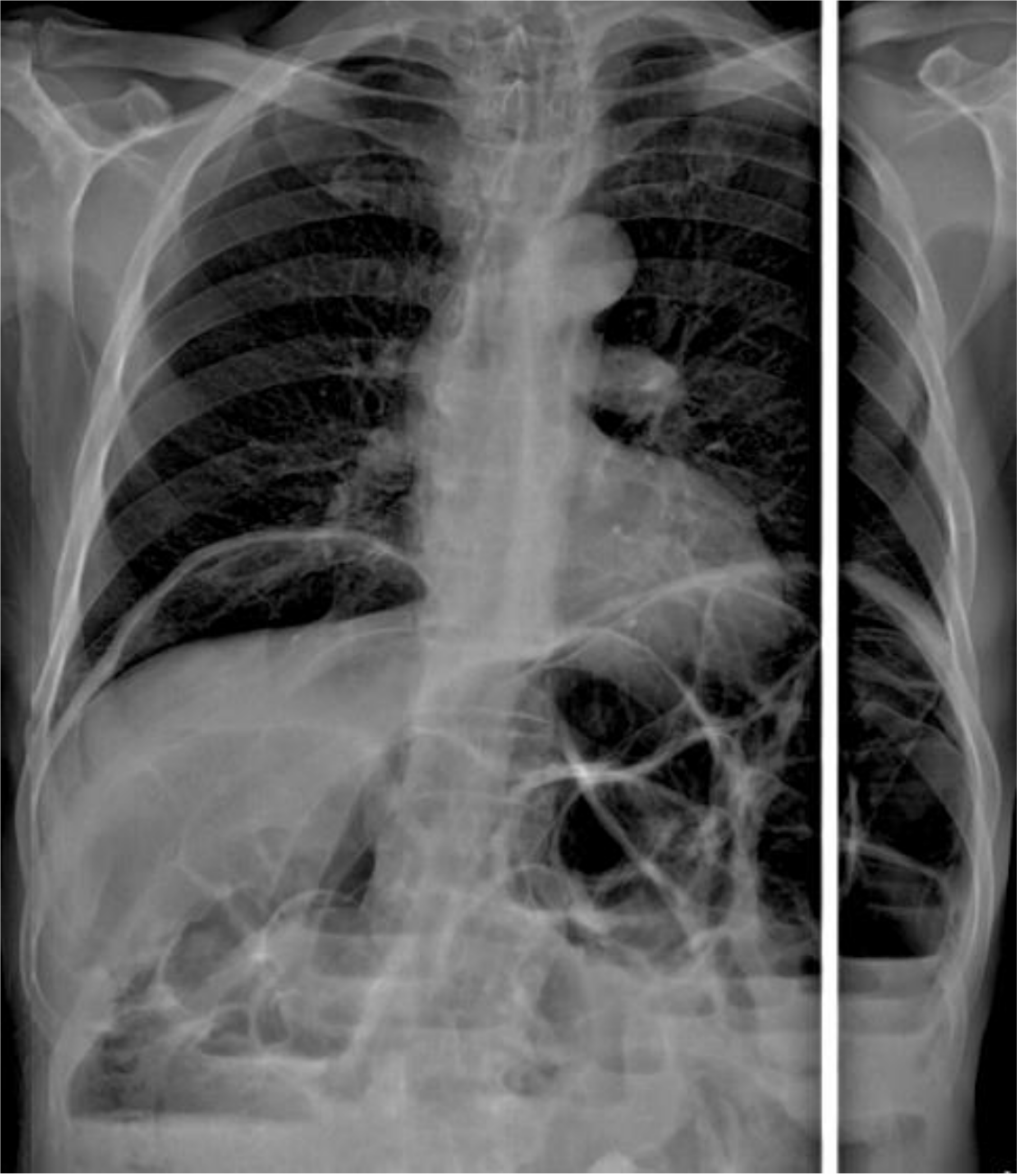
Dilated small bowel, multiple fluid levels and pneumoperitoneum on X-ray.
